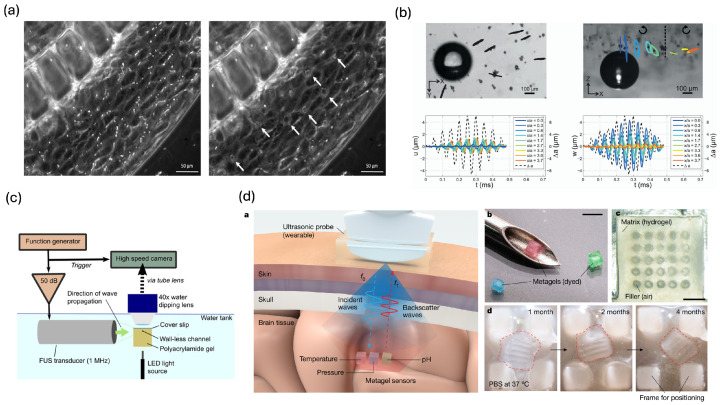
(a) Cavitation-induced endothelial intercellular gaps following microbubble exposure, providing a readout of junctional disruption. Before irradiation, MBs is homogeneously-distributed, dispersed particles; Under ultrasound (US) exposure, the acoustic pressure drives MB aggregation, resulting in evenly spaced clusters (white arrow). Reprinted with permission from reference [148]. (b) Tracer particle motion around an acoustically driven oscillating microbubble, used to quantify microstreaming flows and local shear. Reprinted with permission from reference [150]. (c) Experimental setup for characterizing microbubble oscillation and acoustic streaming in a wall-less polyacrylamide gel channel, enabling sensing of gel-like tissue mechanics. Reprinted with permission from reference [151]. (d) The injectable and biodegradable metagels as wireless intracranial physiology sensors using ultrasound reflection. Scale bars, 2 mm (b), 500 μm (c). Reprinted with permission from reference [152].
